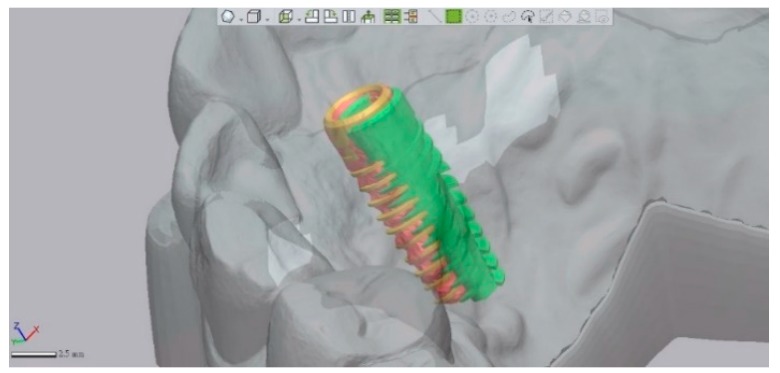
A CAD file (brown) of the implant replaced the ambiguous post-operative CBCT implant image (red) by using the best-fit algorithm, so that the deviation between the planned implant (green) could be more appropriately measured.
